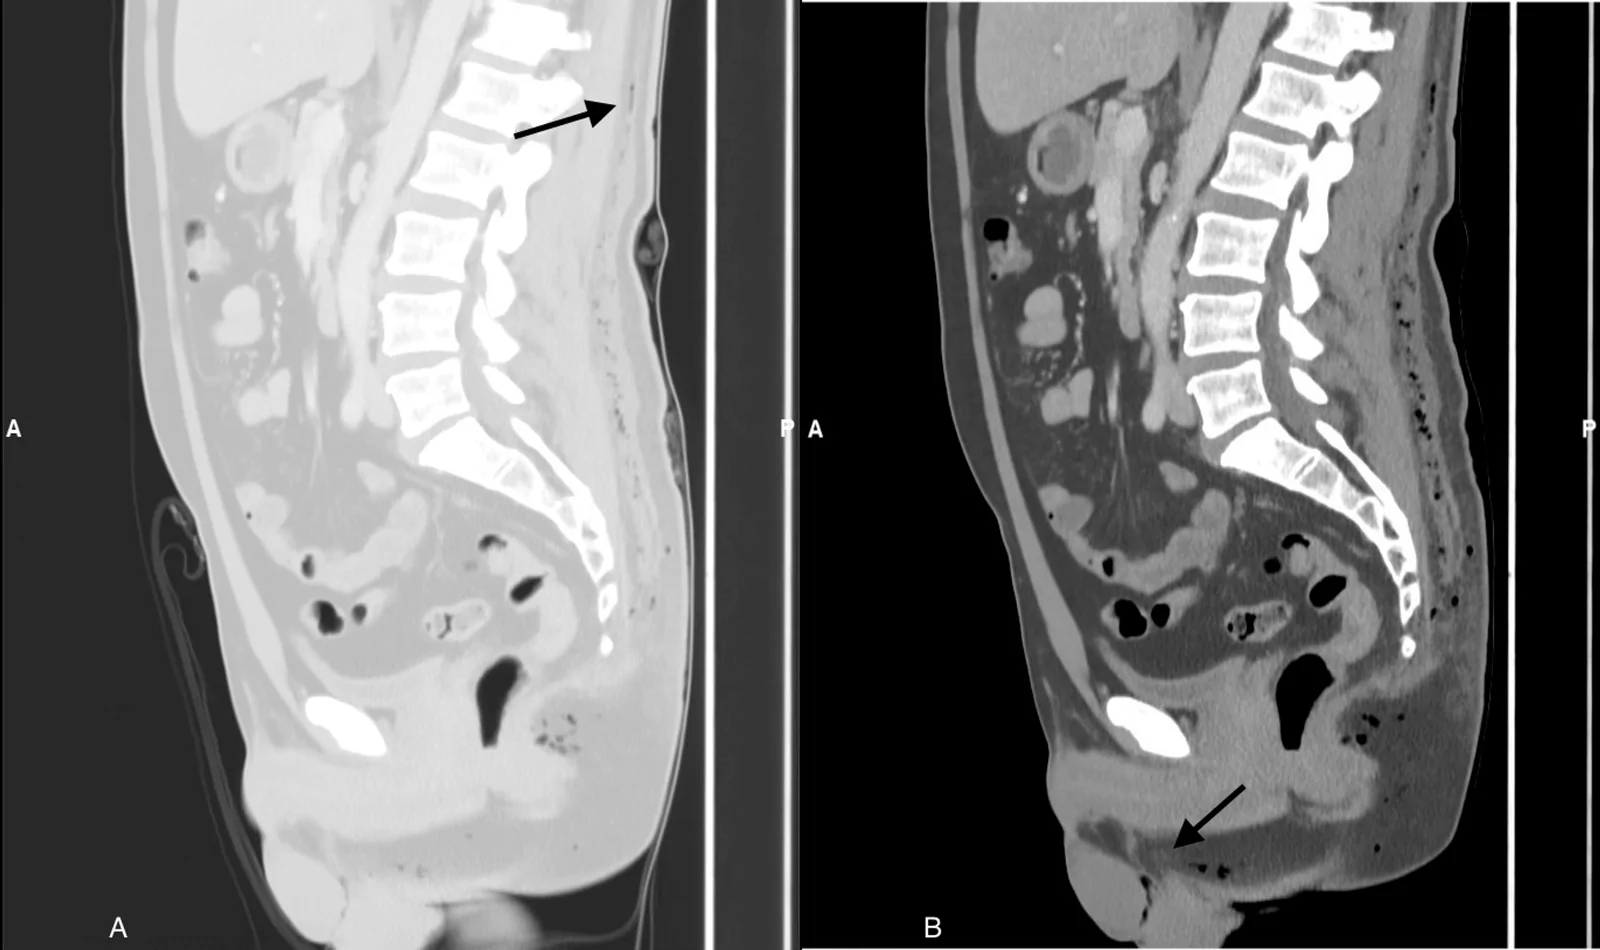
Sagittal view of multislice abdominal CT scan with intravenous contrast, showing subcutaneous air extending from the level of the coccyx up to the T12 vertebra (a), as well as at the perineum and along the right spermatic cord (b).
